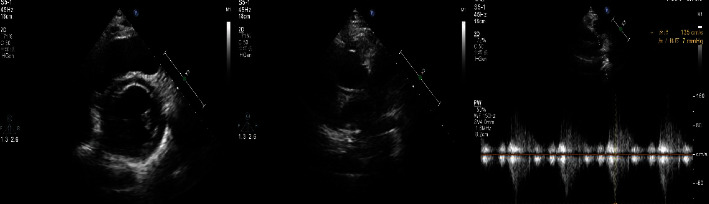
Triplane TDI ultrasound characteristics of patients with SPE (gestational age of 26 weeks).
